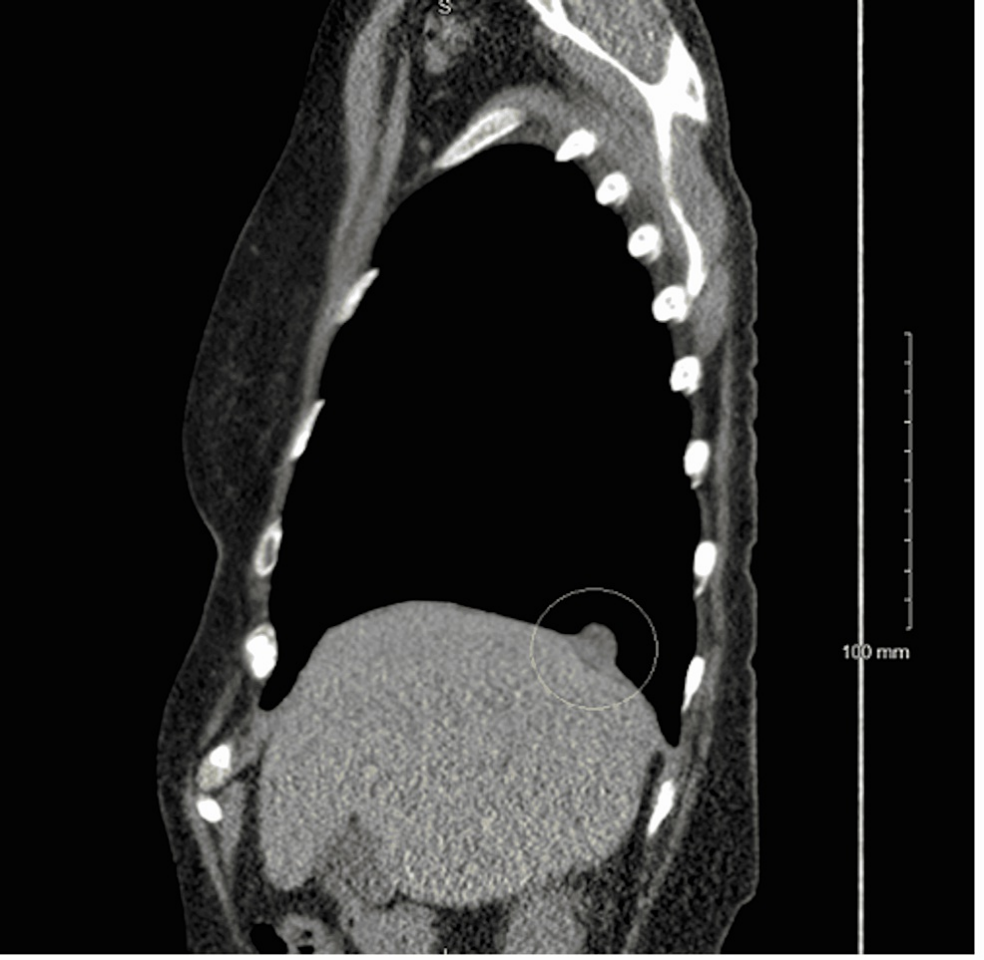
Low dose CT scan lateral view Low dose CT scan lateral view showing the 1.9 cm × 1.8 cm × 1.4 cm mass with smooth margins abutting the right hemidiaphragm
